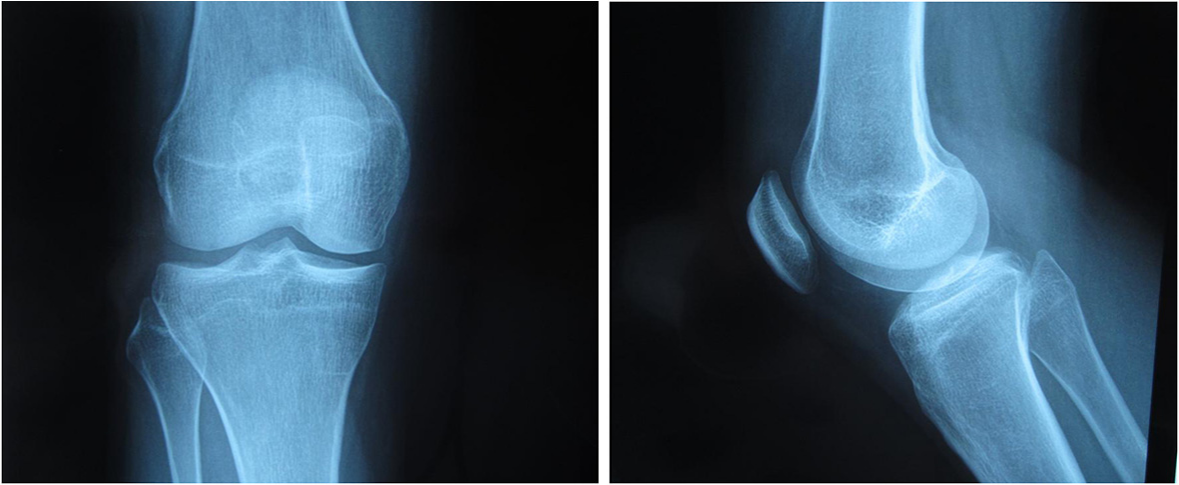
Plain film radiograph of the anterior-posterior and lateral right knee show no abnormalities or loose bodies.
